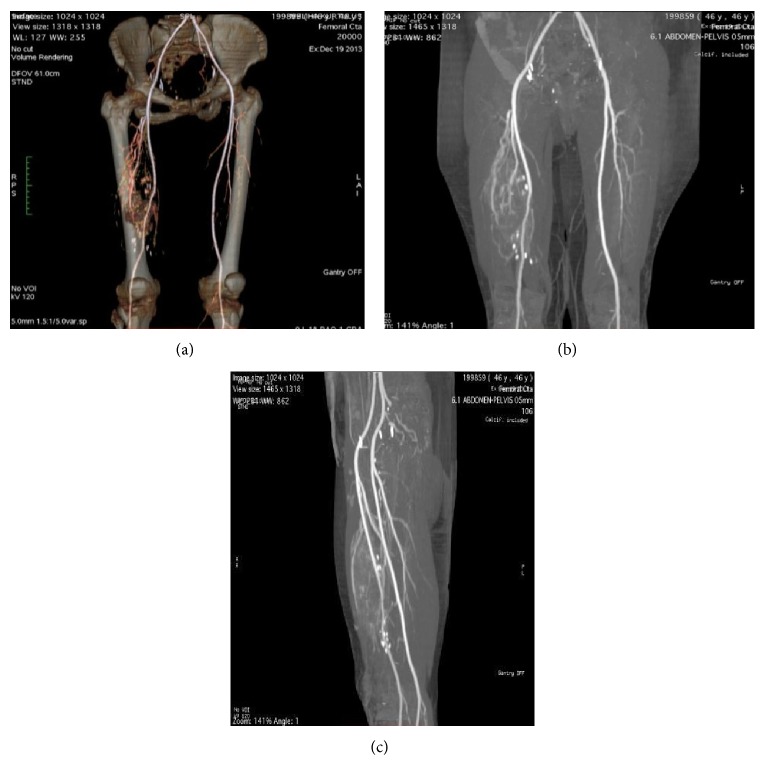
(a) Three-dimensional CT angiography. (b) Coronal plane CT angiography. (c) Sagittal plane CT angiography.
